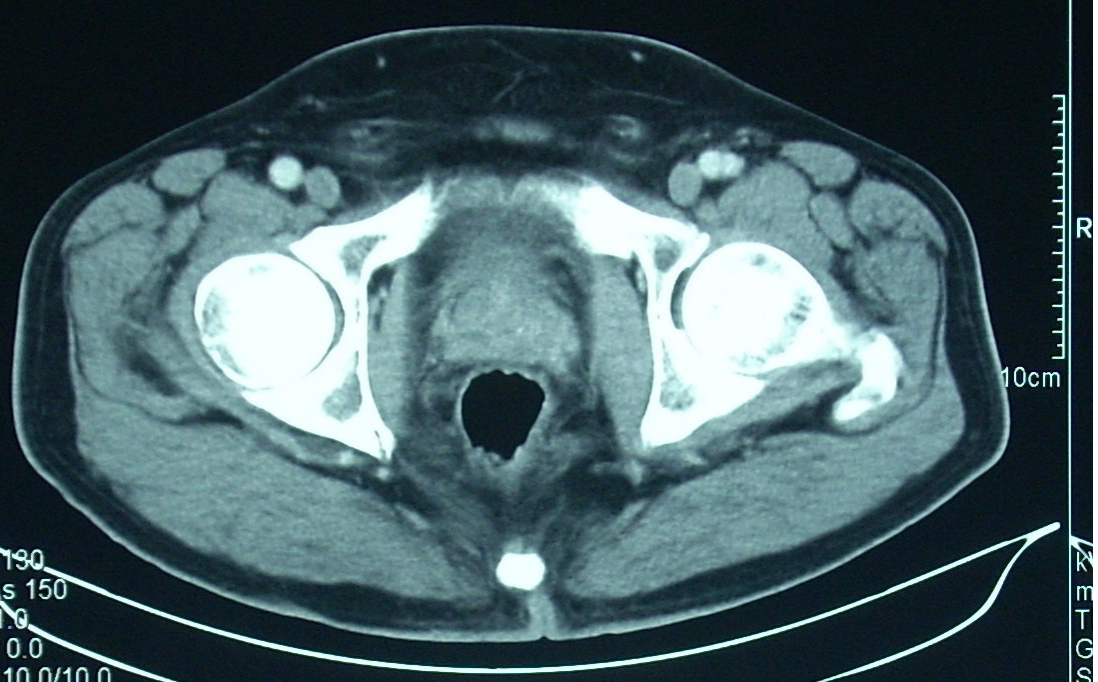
CT reassessment of primary rectal tumour after neoadjuvant treatment.
